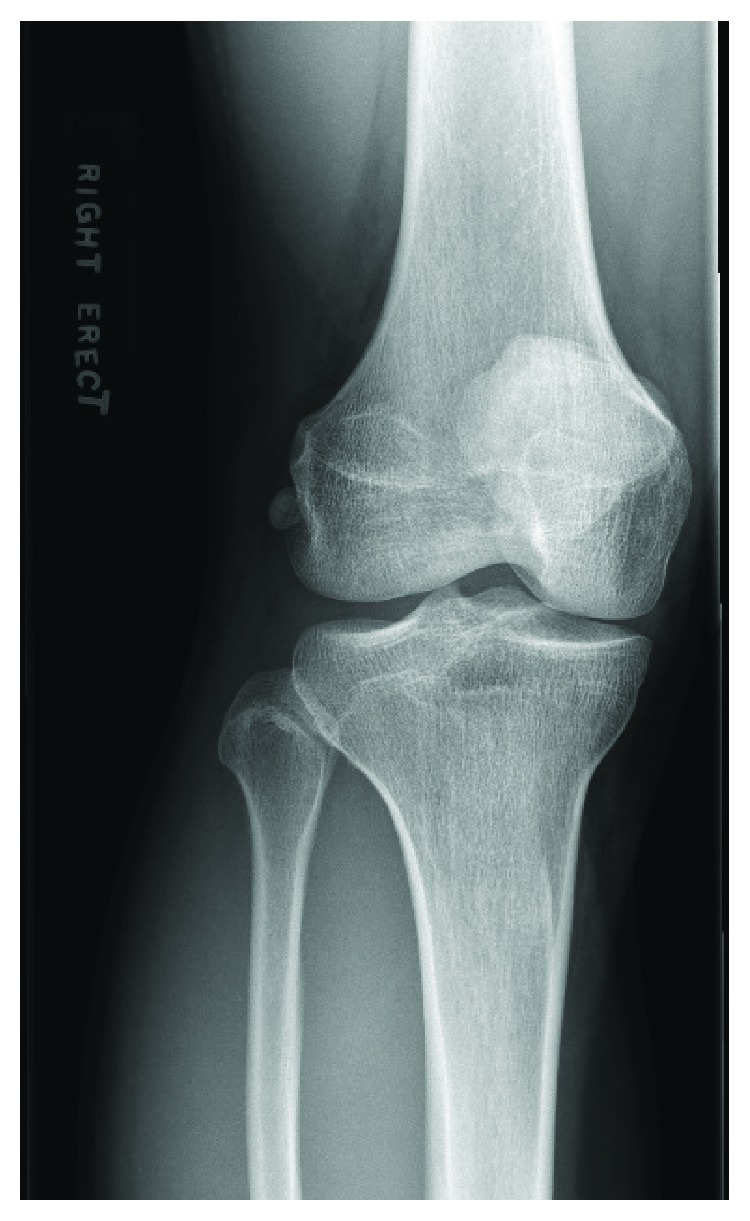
Fabella located lateral out from behind the lateral femur on the AP X-ray.
